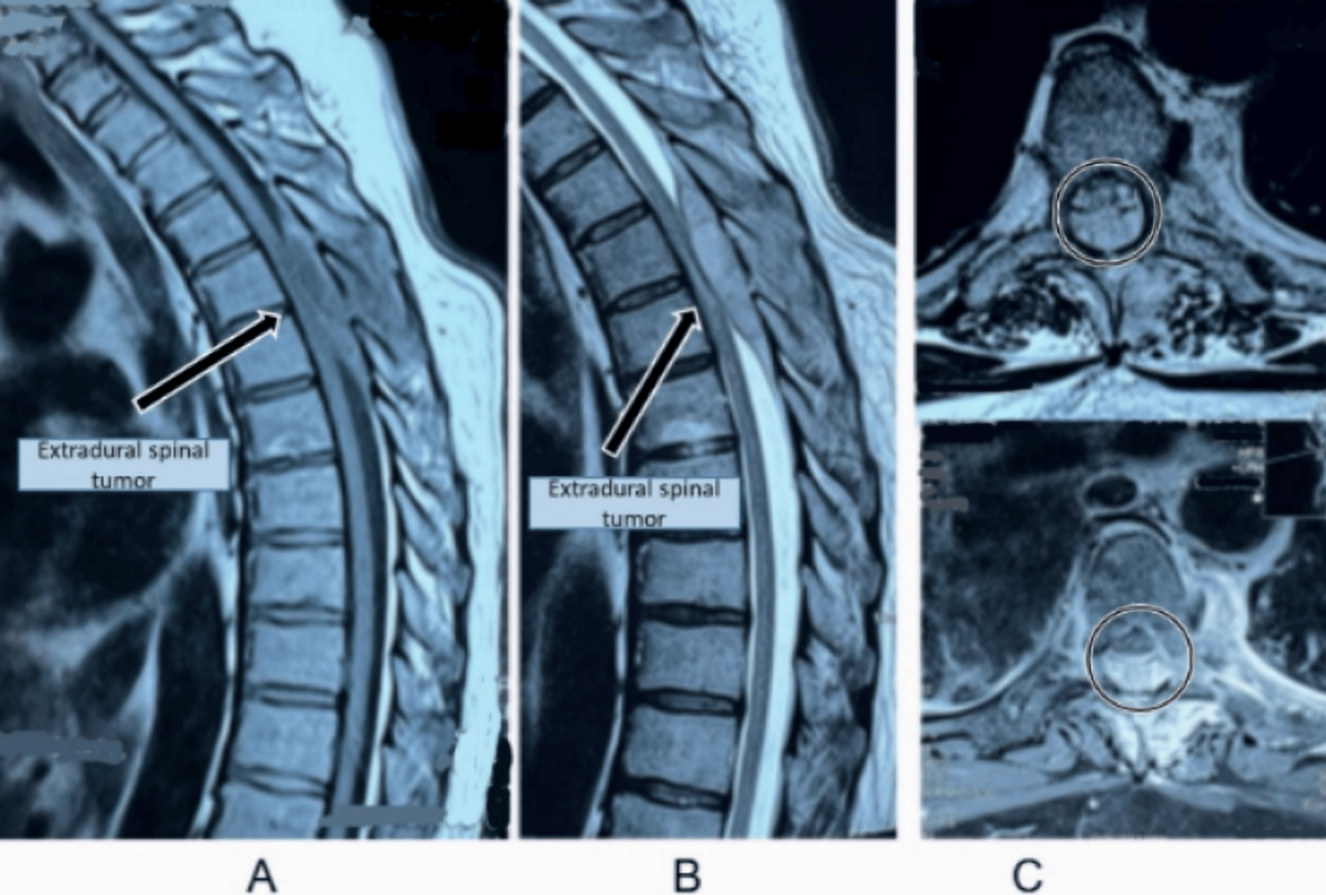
Magnetic resonance imaging of the thoracic spine with gadolinium contrast. (A) Sagittal T1-weighted post-contrast image shows an enhancing extradural spinal tumor (arrow) located between T3 and T6, displacing the spinal cord anteriorly. (B) Sagittal T1-weighted post-contrast sequence confirms the extradural lesion at the mid-thoracic level (T3–T6) with marked mass effect over the spinal cord. (C) Axial T1-weighted post-contrast image at the level of T4–T5 demonstrates the tumor within the spinal canal (circled), producing compression of the dural sac and spinal cord.
